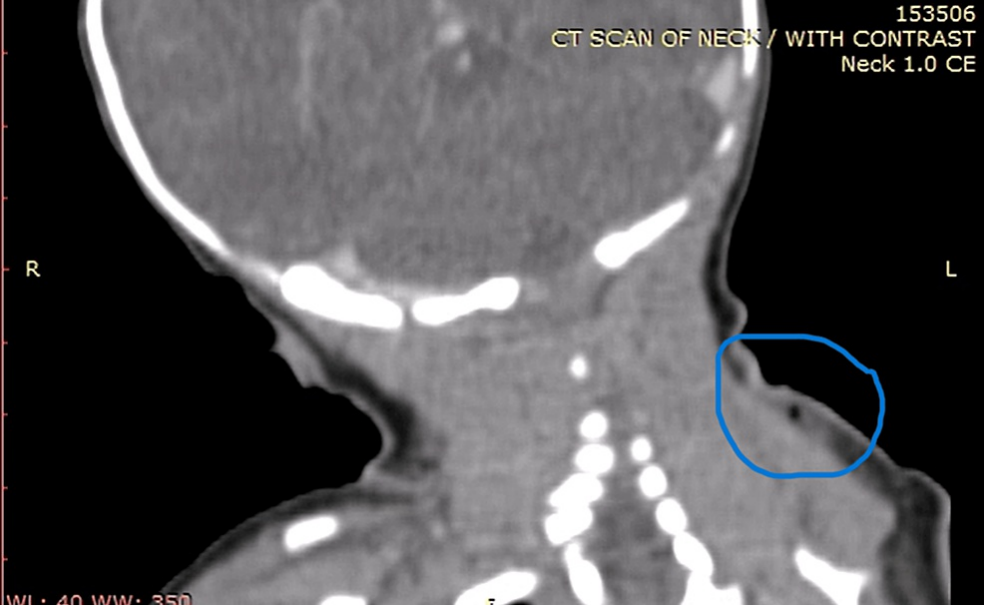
CT scan with Intravenous contrast of the neck showed a 23×13 mm left neck triangle subcutaneous soft tissue edema and gas bubbles favoring cellulitis CT: Computed Tomography
